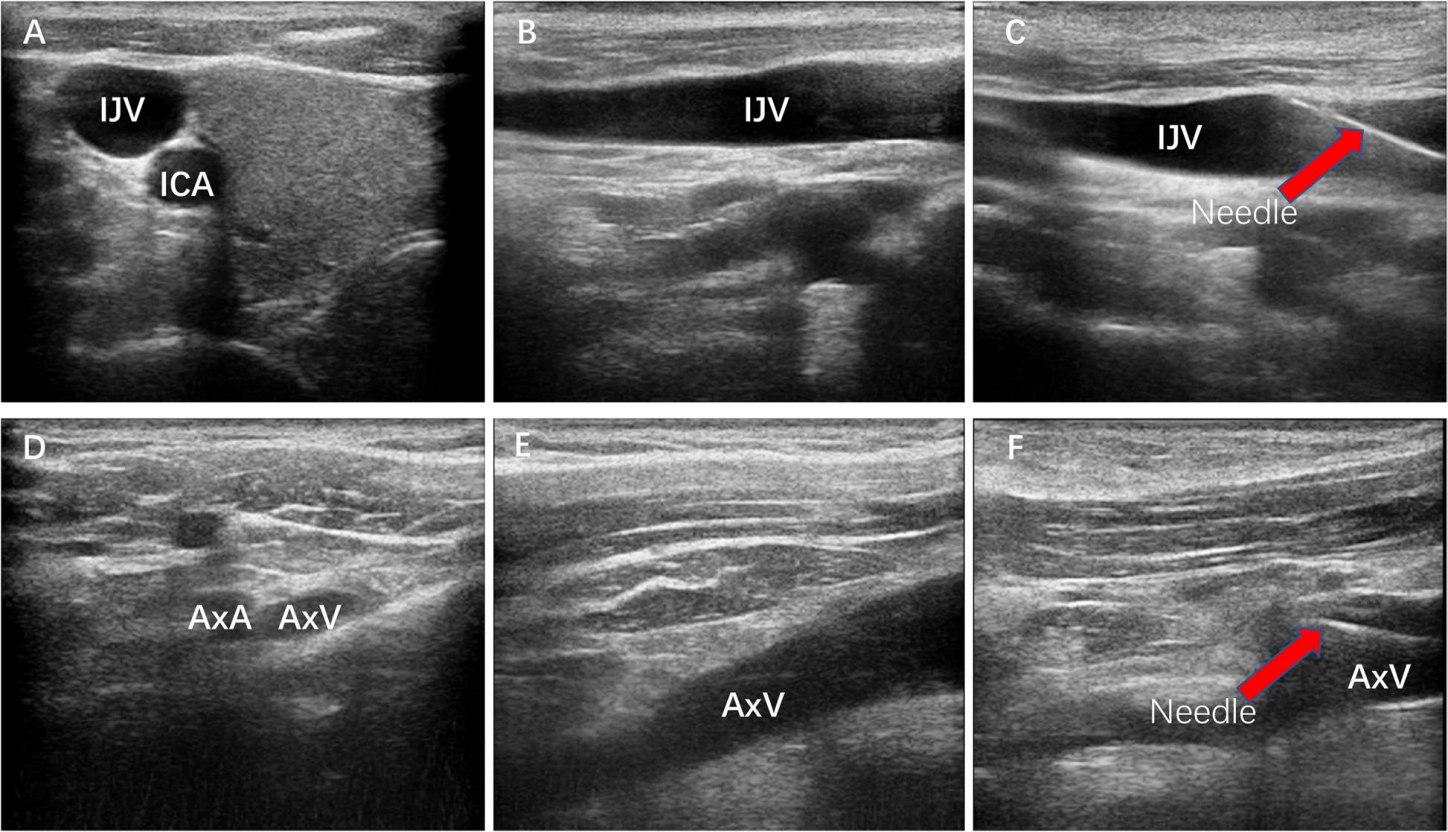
Center venous catheter puncture with ultrasound guidance. A-C Center venous catheter puncture through IJV with ultrasound guidance. D-F Center venous catheter puncture through AxV/SCV with ultrasound guidance. ICV: Internal carotid artery. IJV: Internal jugular vein. AxA: Axillary artery. AxV: Axillary vein. SCV: Subclavian vein
